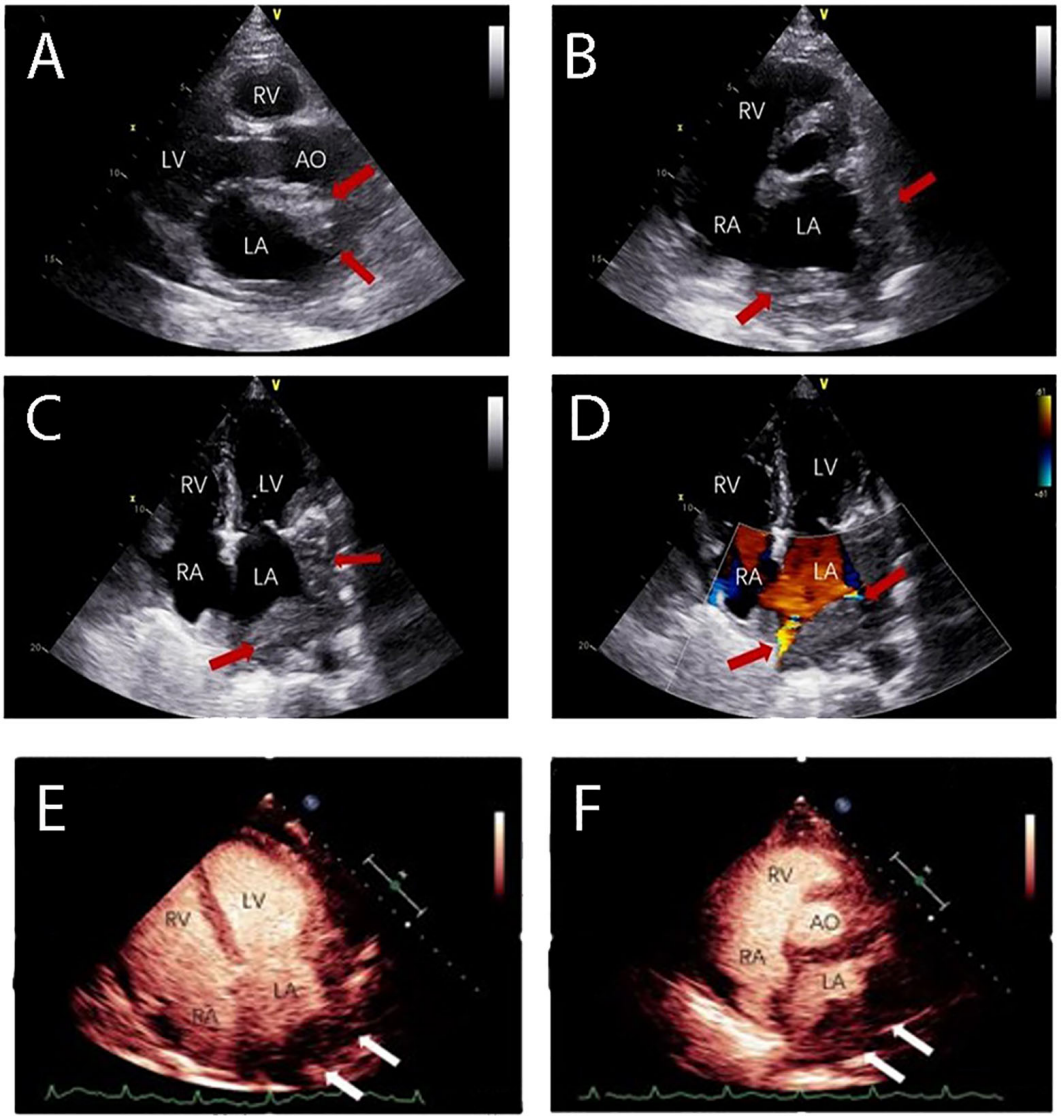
The patient experienced dyspnea and had a refractory bilateral pleural effusion after receiving multi-line chemotherapy. Transthoracic echocardiography showed the heart infiltrated by lymphoma. (A) Long axis section of left ventricle showing a slight hypoechoic mass in the anterior wall of the left atrium. (B) Lateral wall of left atrium and left atrial appendage were infiltrated. (C, D) Four-chamber view showed pulmonary vein infiltration. Cardiac ventricular opacification (CVO): (E, F) An irregular mass was present at the entrance of pulmonary veins in the left atrium with rapid irregular perfusion and complete enhancement. (LV: Left Ventricle; RA: Right Atrium; RV: Right Ventricle; LA: Left Atrium).
